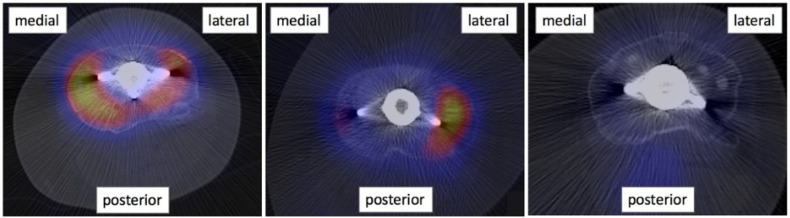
Examples of SPECT/CT images with axial view on the tibial tray. From left: knee with most extended BTU on the medial side of the tibial tray (asymmetrical distribution); knee with most extended BTU on the lateral side of the tibial tray (asymmetrical distribution); no increased BTU detected (symmetrical distribution).
